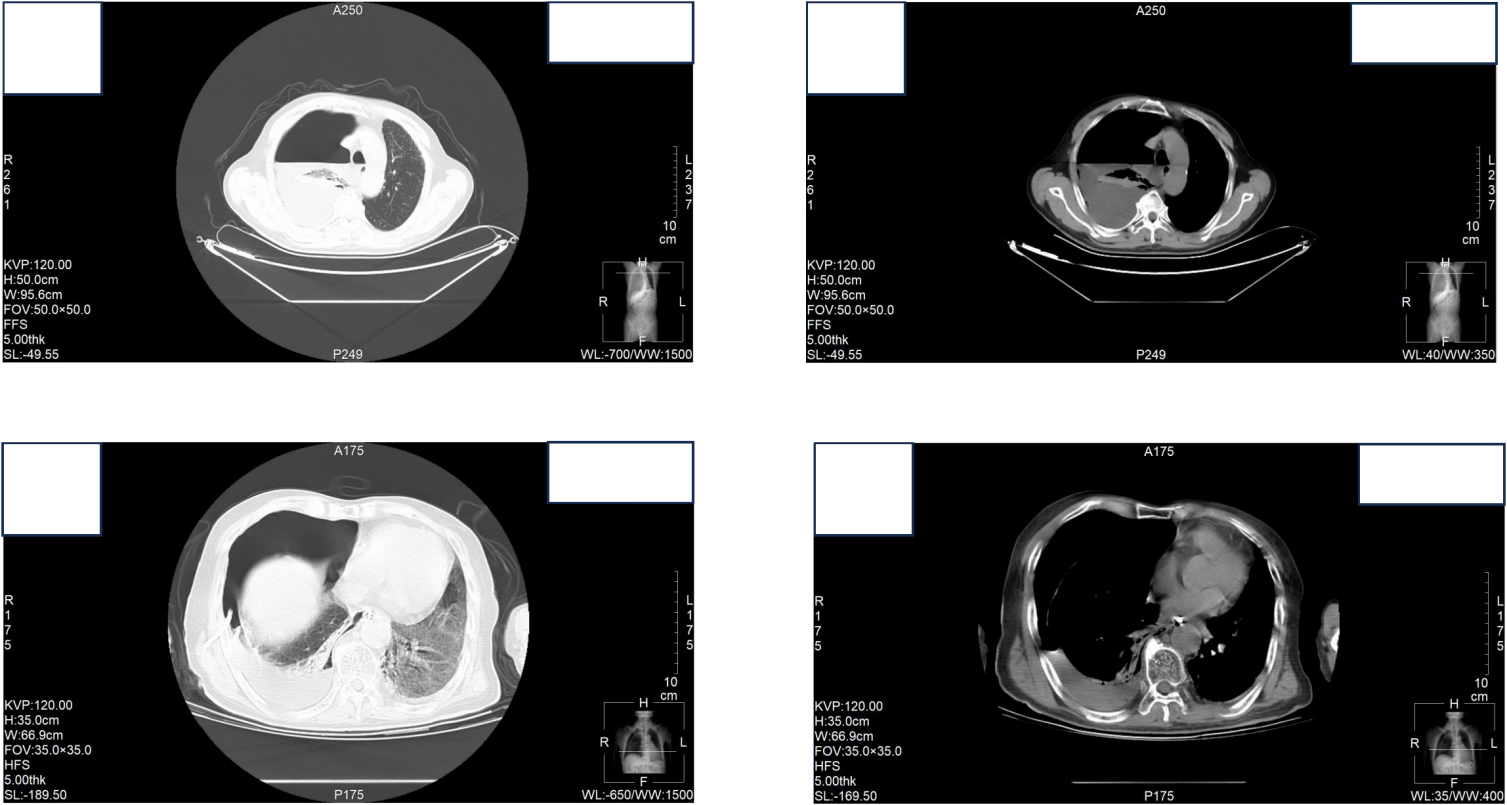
Contrast-enhanced CT scan of the chest demonstrated pleural effusion and hydropneumothorax in the right thoracic cavity.
